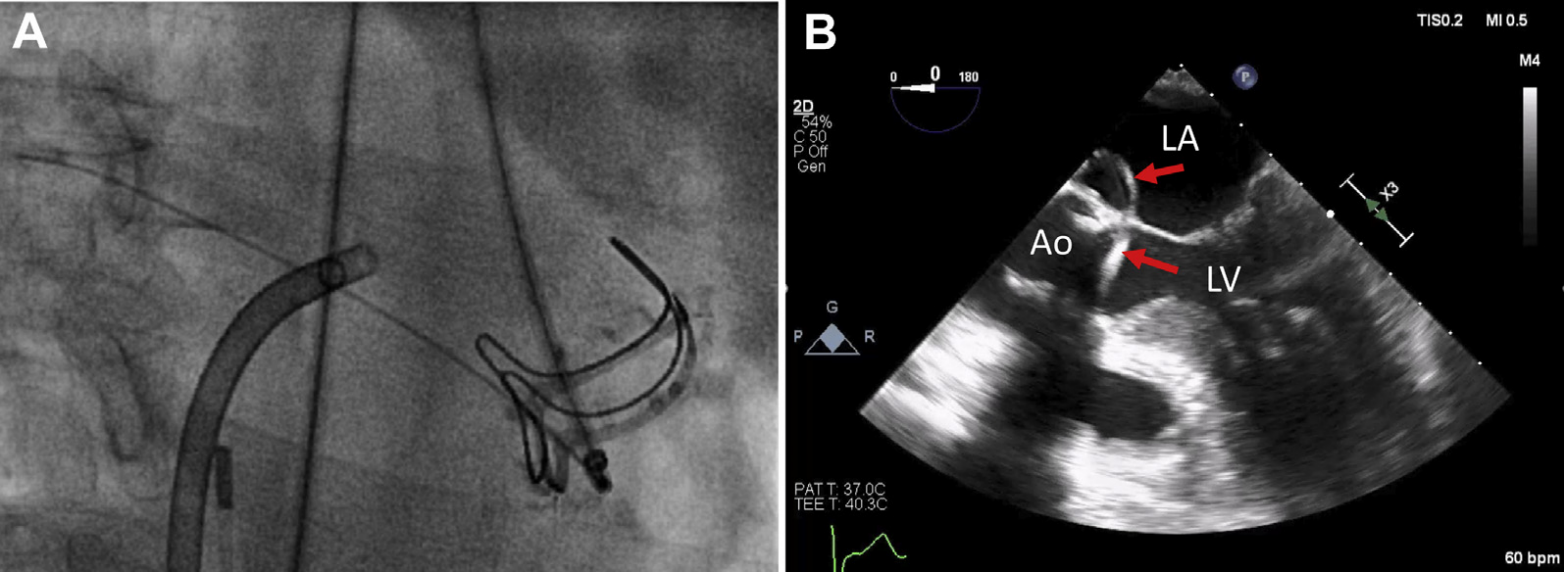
Crossing the Fistula (A) The fistula was crossed from the left ventricular outflow tract into the left atrium and pulmonary vein using a stiff-angled Glidewire under fluoroscopic guidance. (B) The Glidewire (red arrows) can be seen crossing the defect on transesophageal echocardiography. Abbreviations as in Figure 1.
